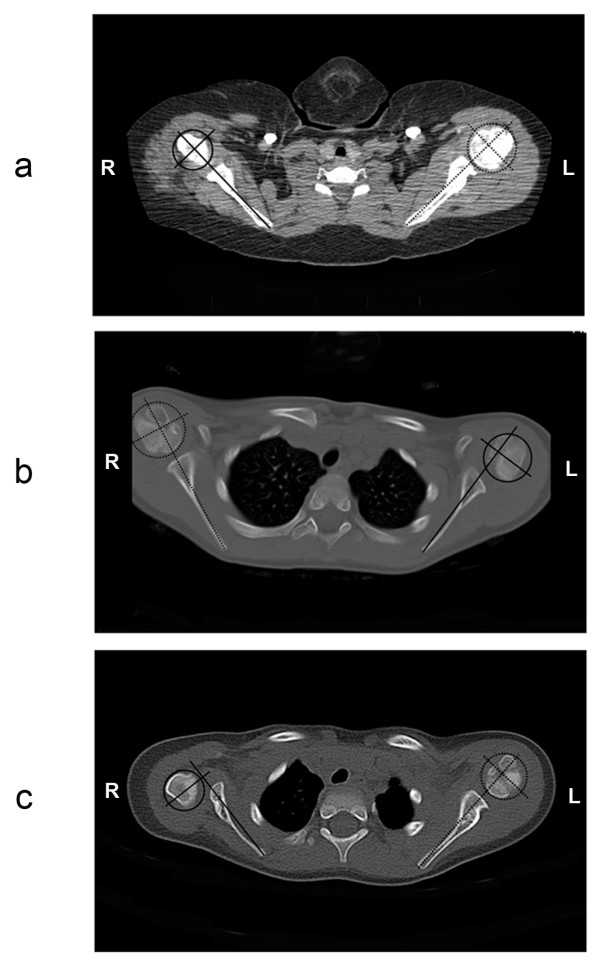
CT Scans of Three Patients with Severe Posterior Subluxation of the Glenohumeral Joint. Bilateral axial CT images of the glenohumeral joints from three OBPI patients with increasing severity of posterior subluxation of the affected humeral head are shown. The difference in the percentage of humeral head posterior subluxation in the affected shoulder (solid lines) compared to the normal shoulder (dashed lines) was measured as 3.9% (a), 15.7% (b), and 53% (c) using the indicated lines as described previously [14].
